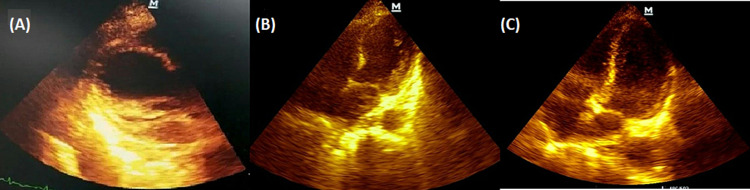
Echocardiogram showing (A) apical ballooning, parasternal long-axis view, and (B) improvement of apical ballooning, apical four-chamber view. (C) Echocardiogram of day 14, apical four-chamber view
